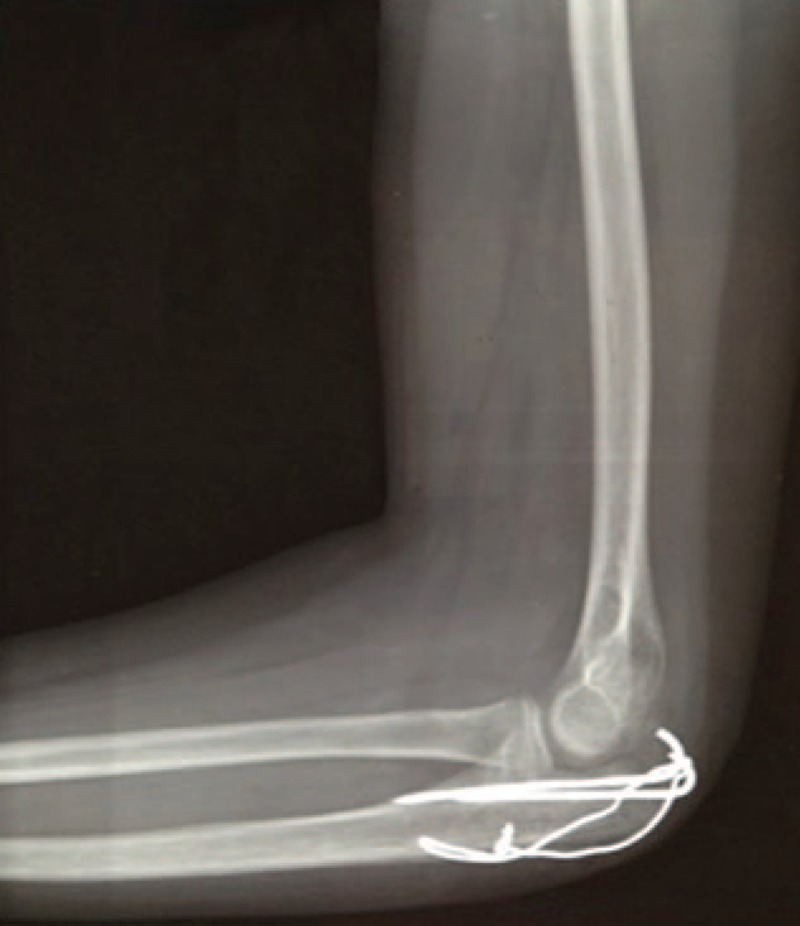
X-rays 1 year after surgery, showing the excellent outcome in fracture healing.
